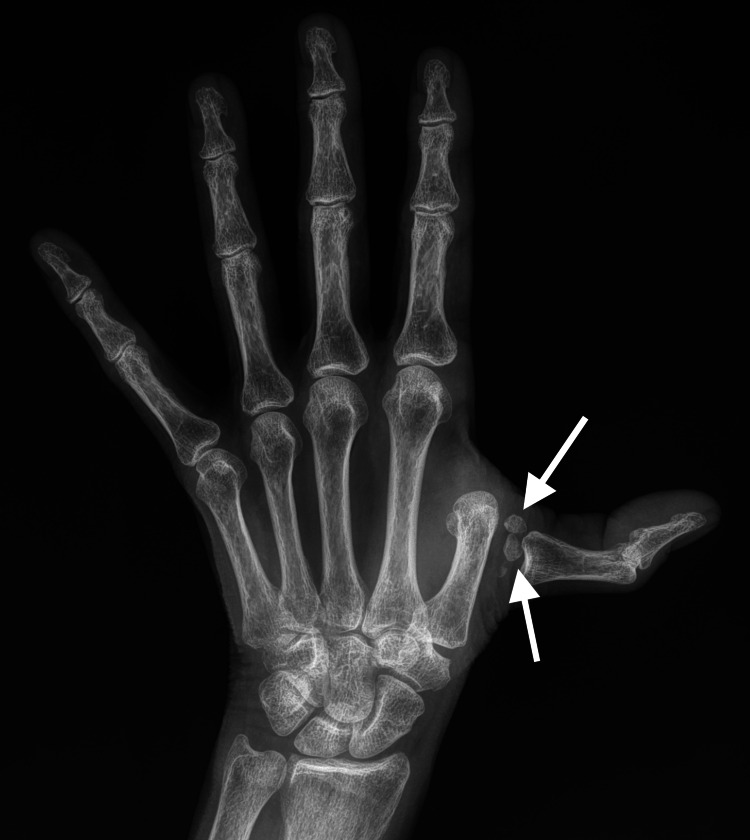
Radiograph of the patient's left hand demonstrating a dorsally displaced dislocation of the first metacarpophalangeal joint with interposition of multiple sesamoid bones. The white arrows indicate the sesamoid bones lodged at the base of the dislocated first proximal phalanx.
